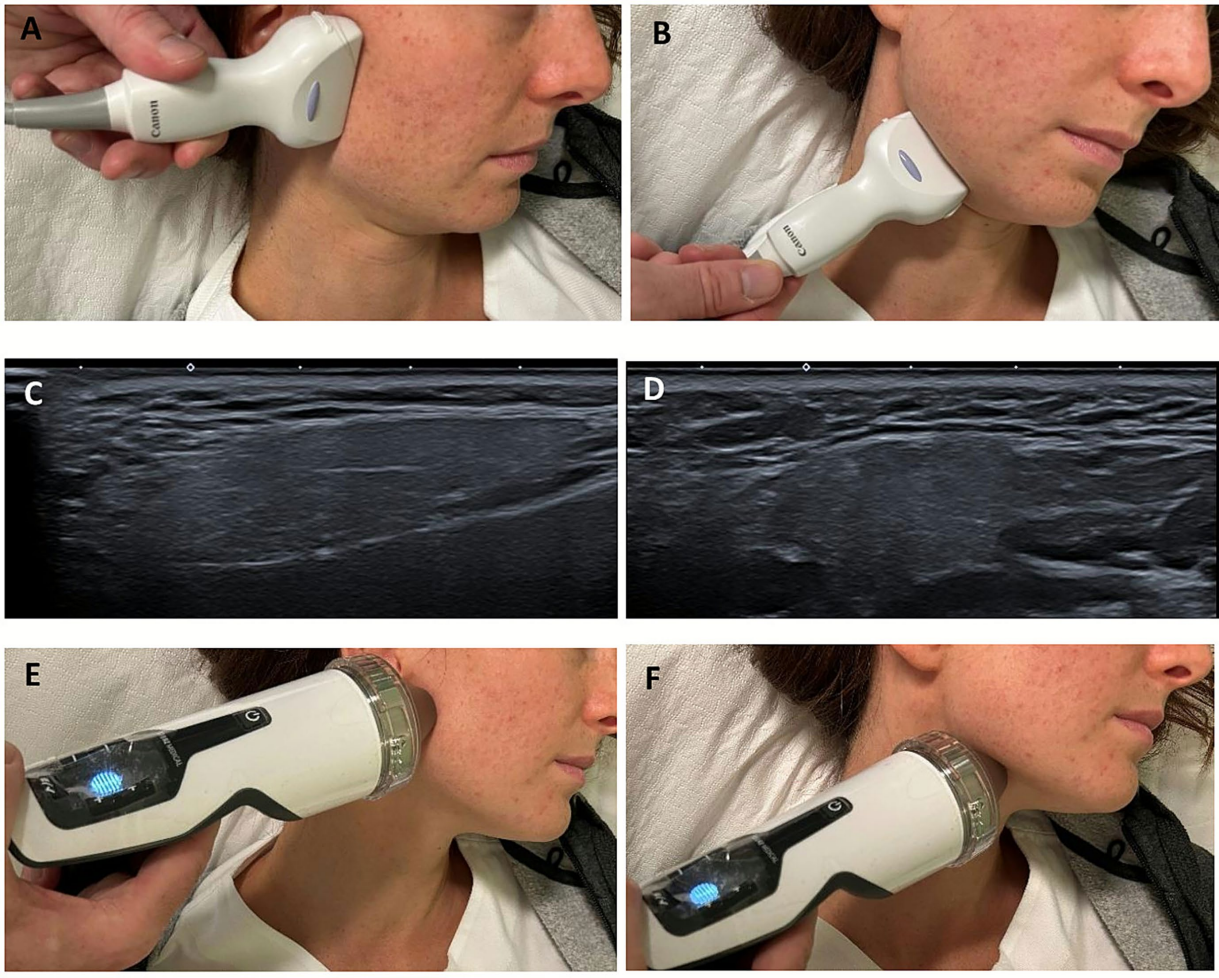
Clinical photographs showing the positioning of the ultrasound probe and the ESWT applicator the over the parotid and submandibular regions (A,B,E,F). Corresponding ultrasound images confirming correct probe placement and real-time visualization of the targeted glandular tissue, parotid (C) and submandibular (D), respectively.
